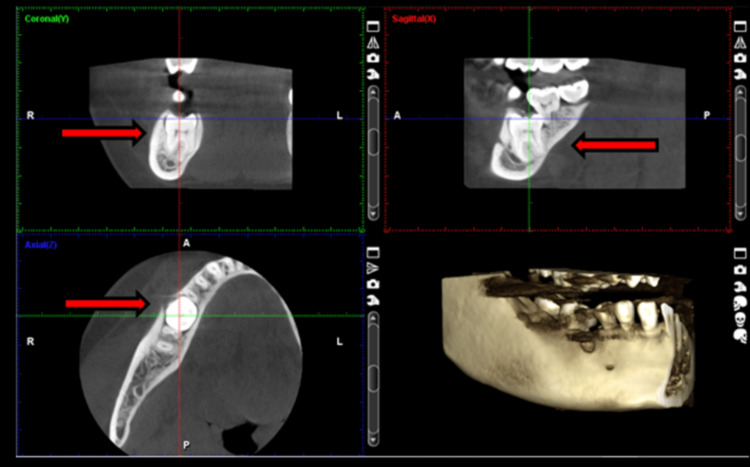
Cone beam computed tomography (CBCT) image of an impacted mandibular first molar
